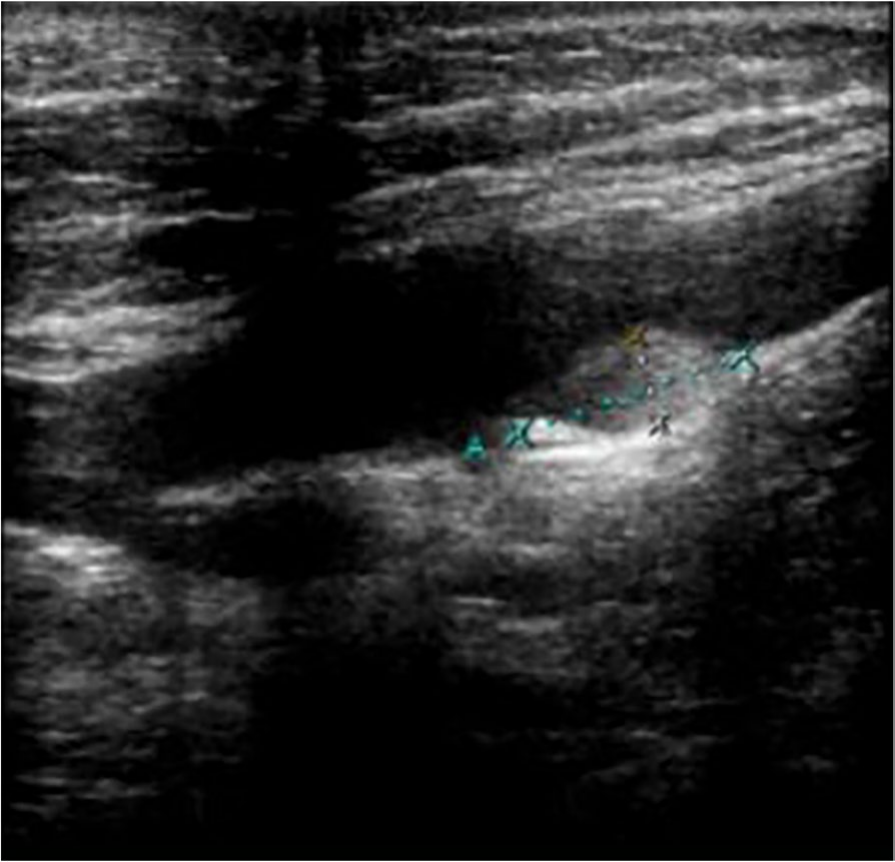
The axillary vein thrombosis on the longitudinal section of the ultrasound
